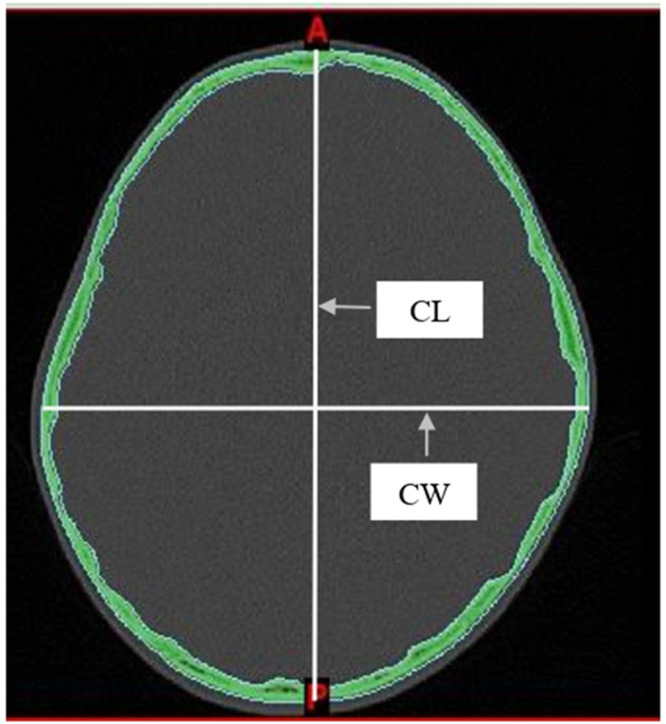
Measurements from axial CT scan. CL, Cephalic Length, CW, Cephalic Width. Cephalic index = CW/CL × 100.
